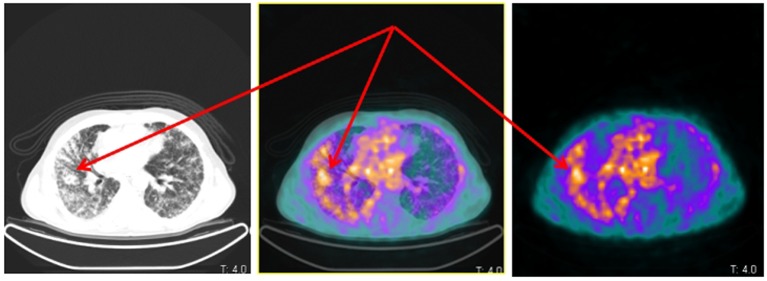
^68^Ga-DOTANOC transaxial PET/CT (left CT attenuation correction, middle fused PET/CT, right PET only) showing increased uptake of the radiopharmaceutical in the left sovra-acetabular region corresponding to a mixed (predominantly lytic) skeletal metastasis (arrows).
